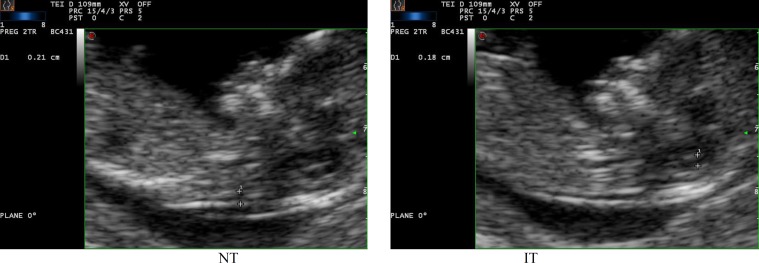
Nuchal Translucency (NT) and Intracranial Translucency (IT) length was measured by ultrasound
